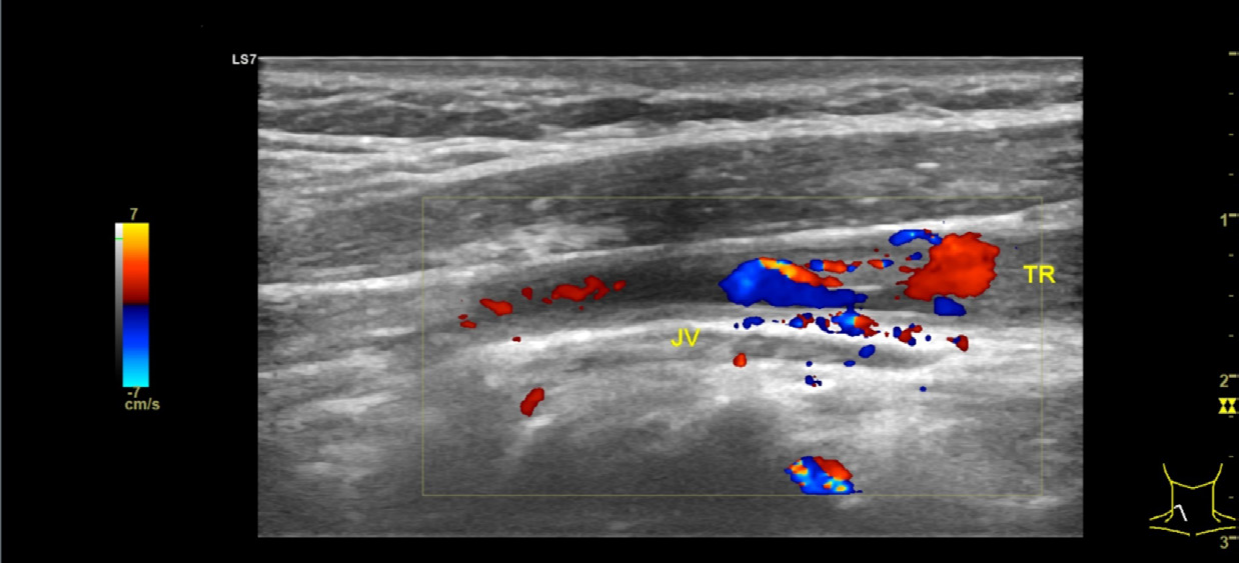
Follow up neck ultrasound revealed partially restored blood flow.
